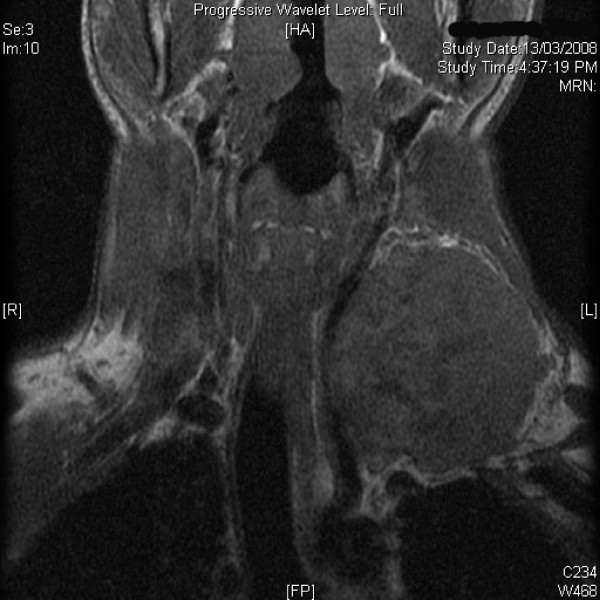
T1 W magnetic resonance image of the neck (coronal section) showing a mass in infrahyoid neck which is isointense to hypointense to muscle.
